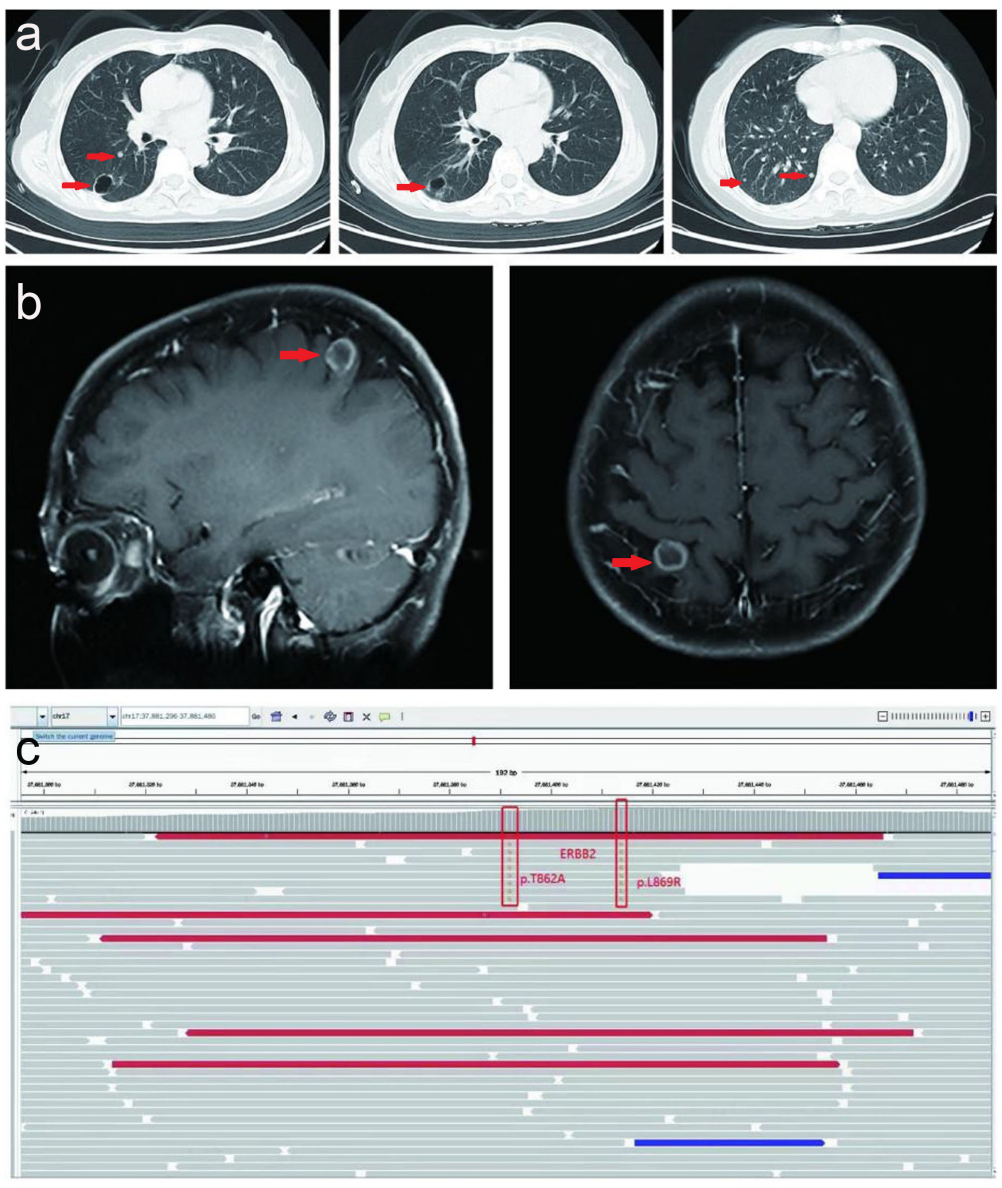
Serial CT scans and MRI. (A) Serial CT scans show multiple metastatic lung lesions were slightly increased after a combination of gemcitabine (1.4g day 1 and day 8) plus anlotinib (8mg, twice a day, day1-14) plus carrelizumab (200mg, day 1) for 2 cycles in June 2022. (B) MRI indicated and one new brain metastatic lesion was observed in June 2022. (C) NGS result of circulating tumor DNA before fifth-line treatment in August 2022.
